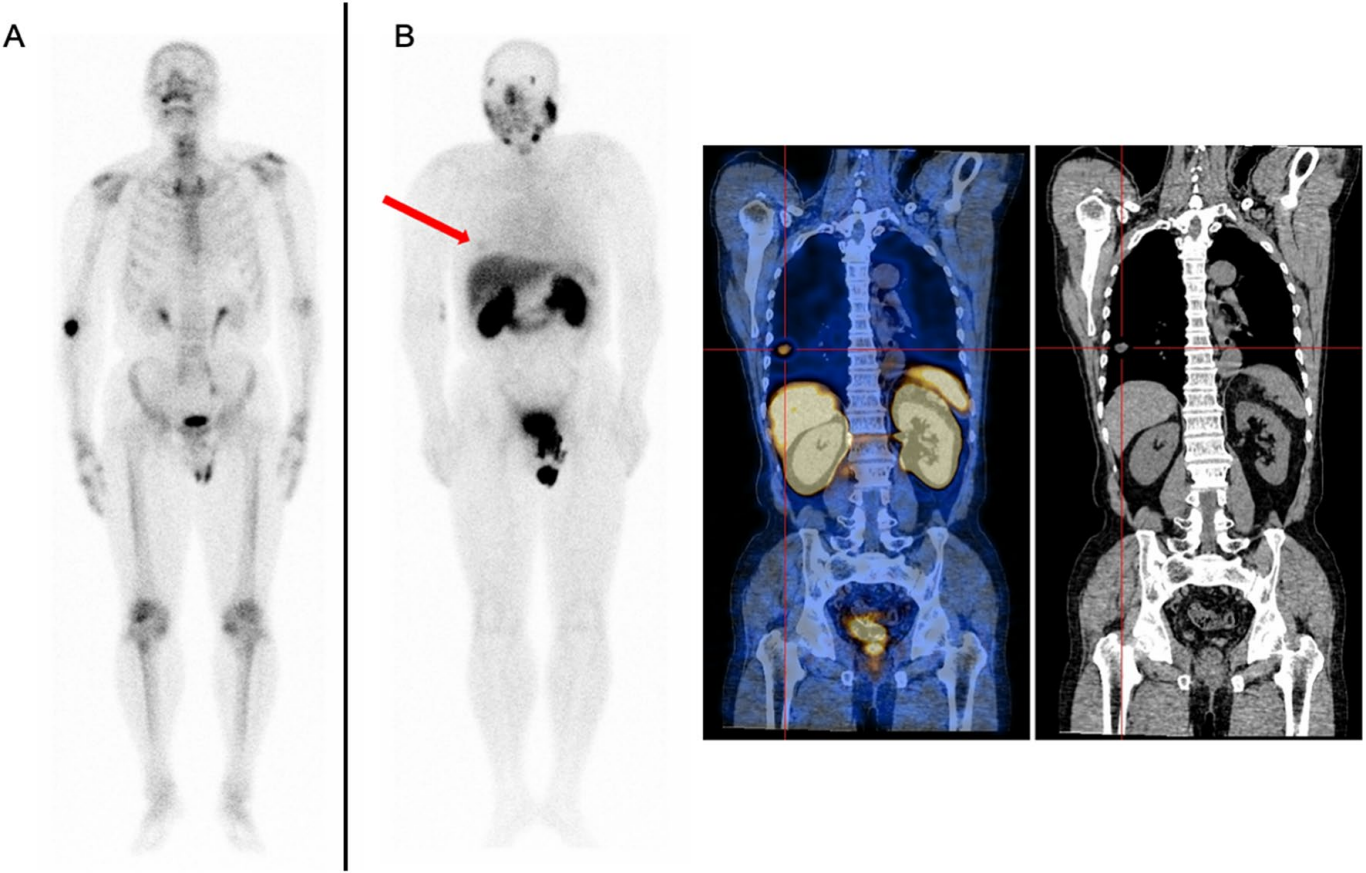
A 66-year-old patient with a BR of PCa. The Gleason score was 7, and the PSA level was 3.14 ng/mL. (A): Whole-body planar ^99m^Tc-MDP. (B): Whole-body planar ^99m^Tc-PSMA and coronal SPECT/CT. ^99m^Tc-PSMA SPECT/CT helped identify lung metastasis (B, red arrow and cross).
